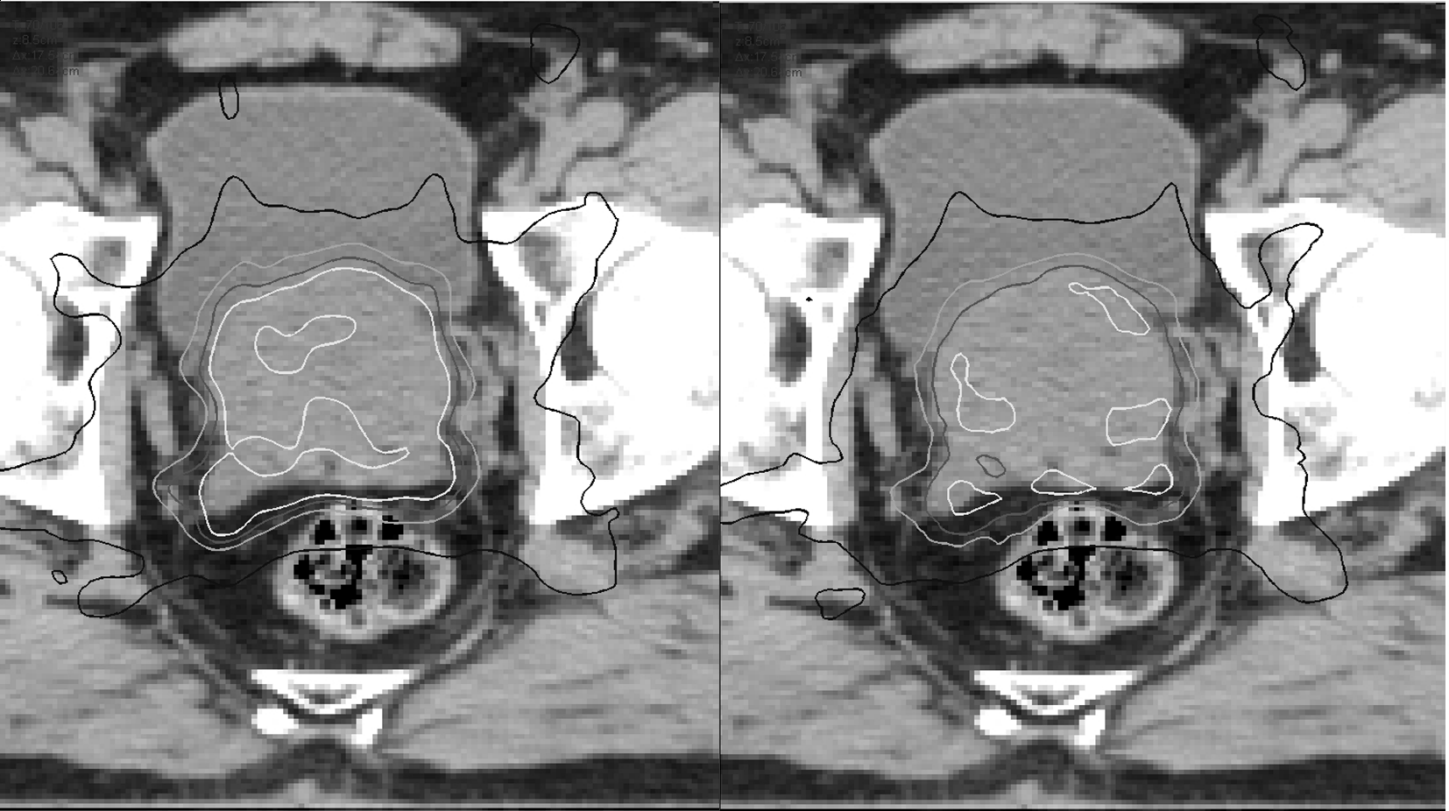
Planned vs. cumulative delivered dose for a patient with large interfraction variation. The planned (left) and cumulative (right) dose distributions are overlaid onto the planning CT. Isodose lines are 105% (white), 100% (dark gray), 90% (light gray), and 67% (black) of the prescription dose.
